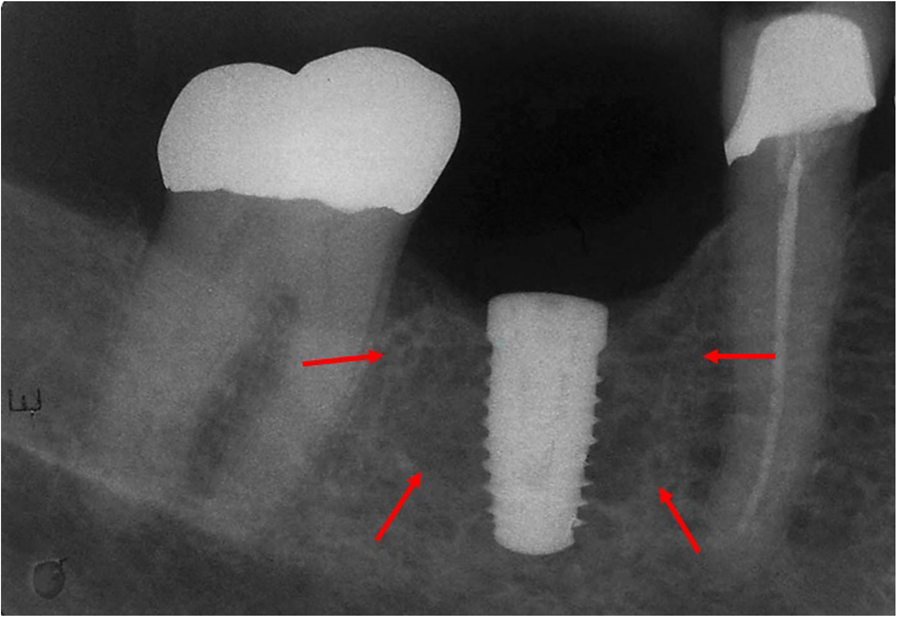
The periapical radiograph revealed the presence of an extensive and poorly circumscribed osteoporotic area around the proximal implant
